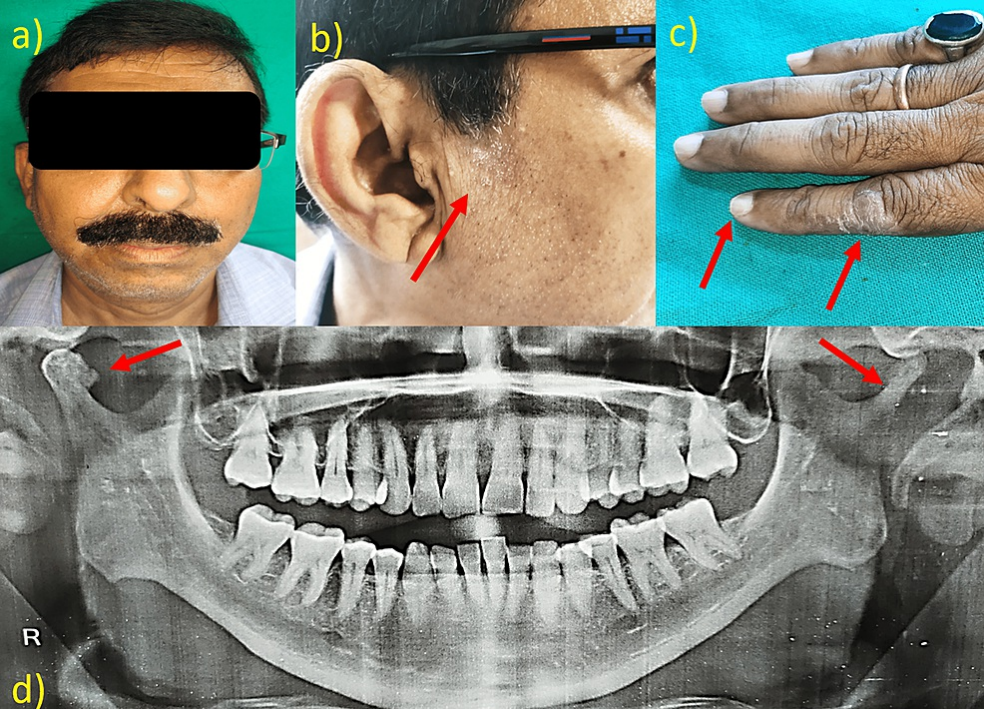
a) No significant asymmetry, b) presence of psoriatic patch over the facial region, c) involvement of nails and fingers, d) the panoramic radiograph reveals bony erosion with osteophyte in relation to the right condyle while a sharpened pencil appearance in relation to the left TMJ TMJ: temporomandibular joint
